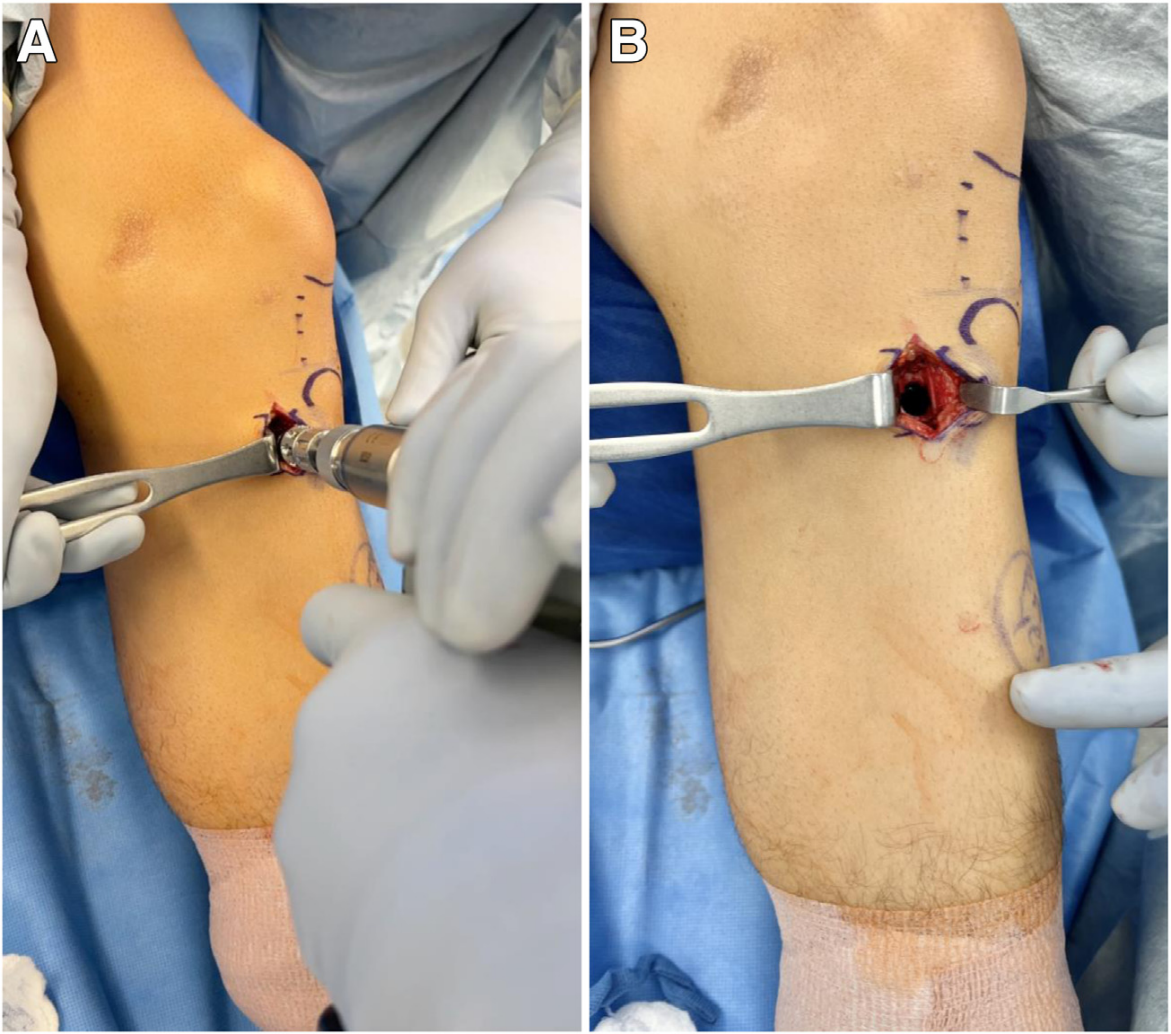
(A) The OsteoAuger is used to ream and harvest 8 to 10 cm^3^ of bone graft in the proximal tibia. This amount is standardized based on the amount of subchondral bone involvement on magnetic resonance imaging and can be augmented with allograft. (B) The resulting bone void is shown and is packed with cancellous allograft chips.
